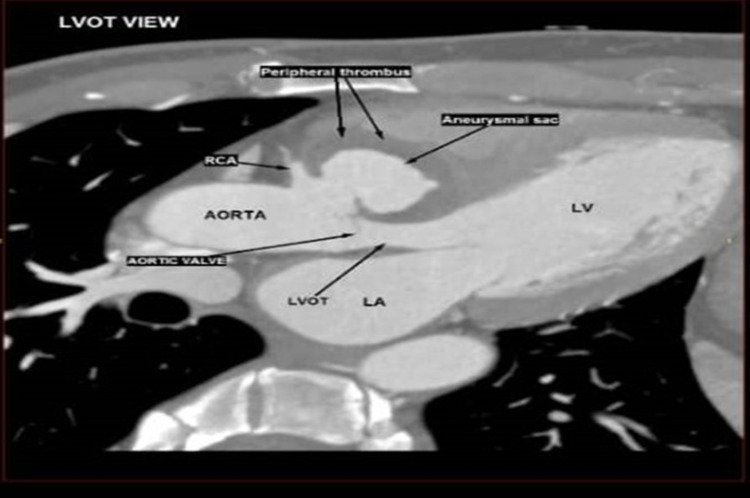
Cardiac CT showing the aneurysm sac with compression effect to the left ventricular outflow tract. RCA: right coronary artery; LV: left ventricle; LVOT: left ventricular outflow tract
